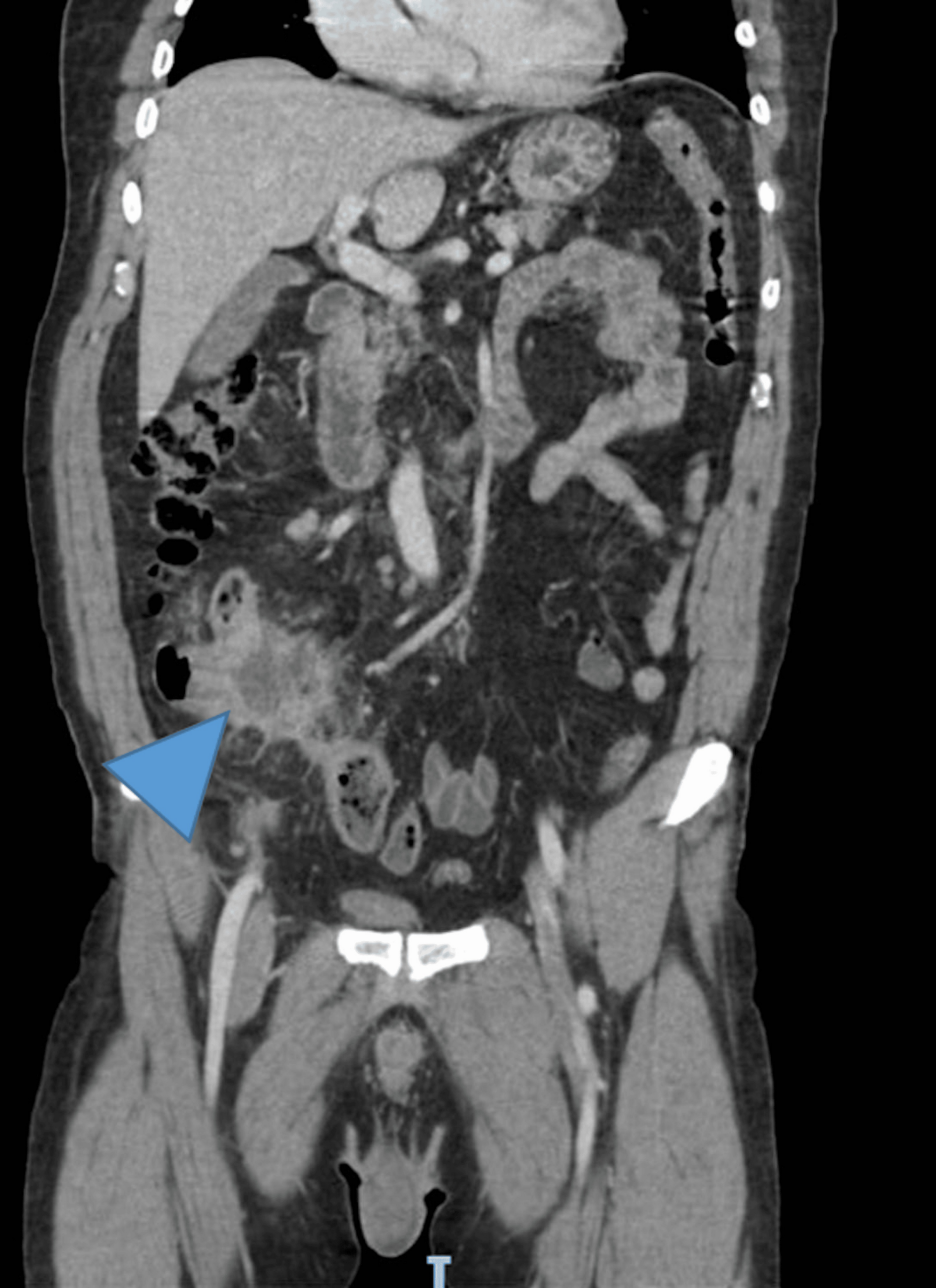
Periappendicular abscess. CT scan coronal view showing periappendicular abscess (arrowhead).
